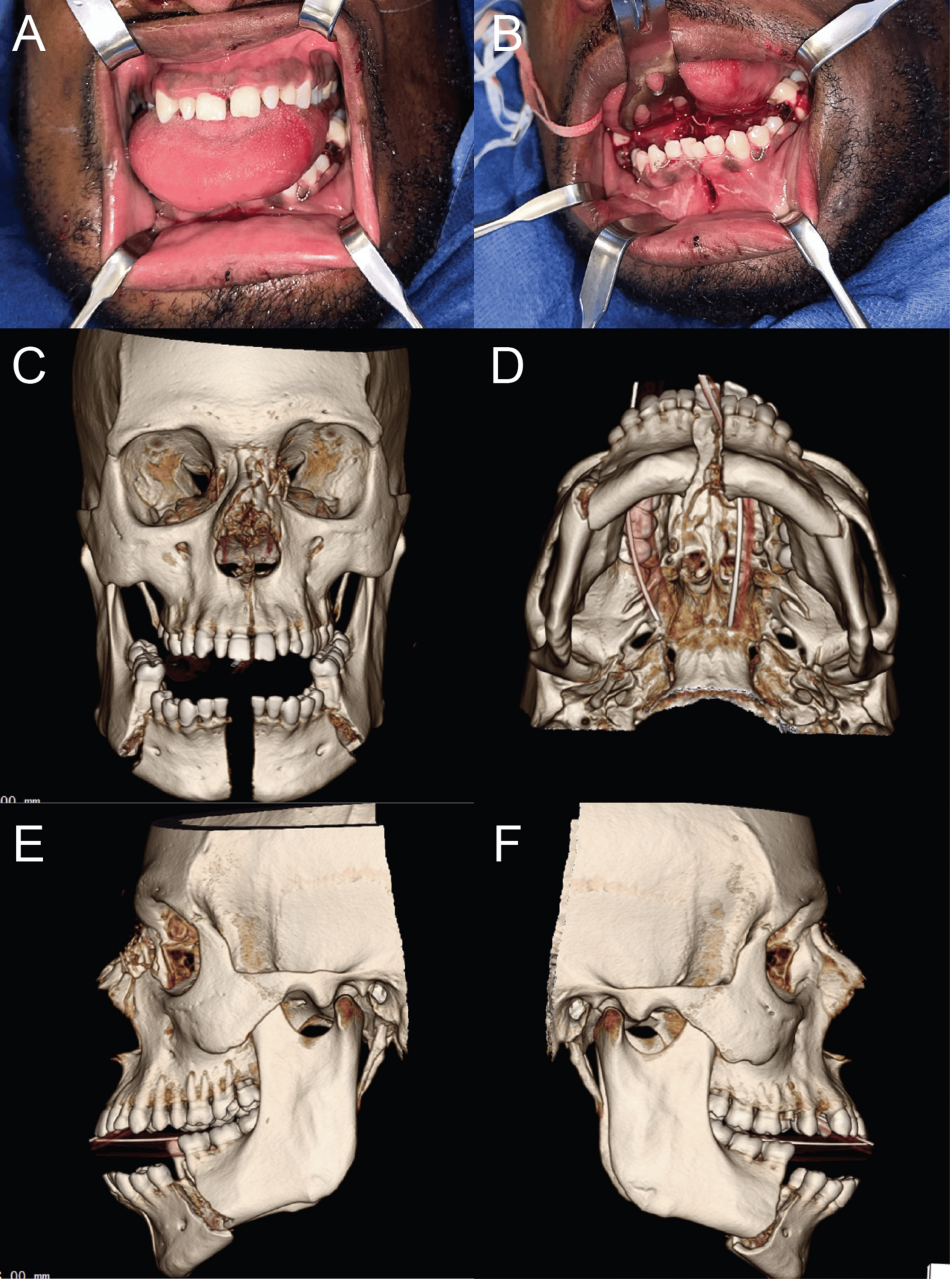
Preoperative presentation and 3D reconstruction of maxillofacial computed tomography scan. (A,B) Clinical photographs showing the fracture sites from two different angles. 3D reconstructions of full-face computed tomography scans showing the mandibular fractures from the (C) frontal, (D) coronal, (E) left sagittal, and (F) right sagittal views
